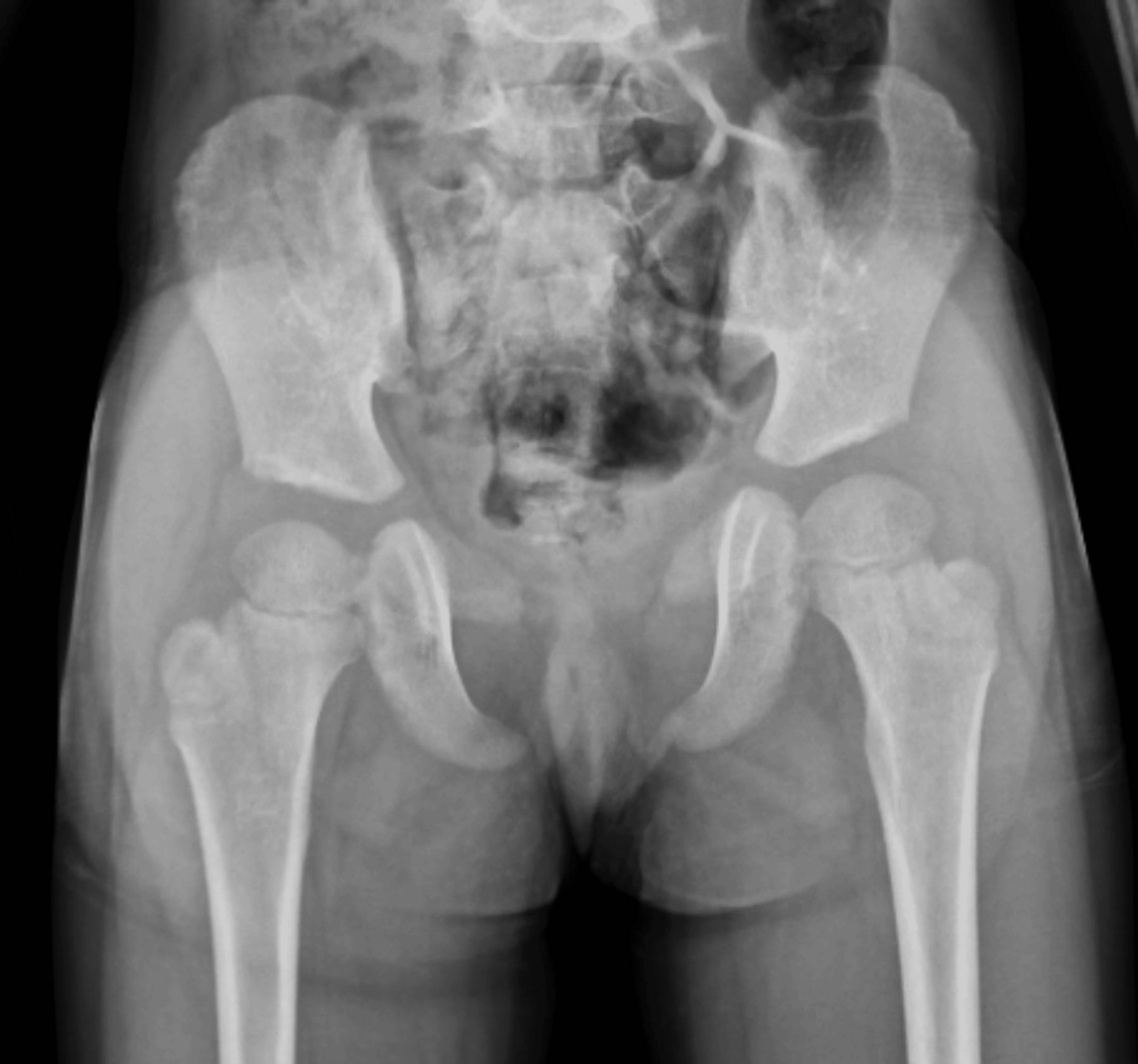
Frontal pelvic radiograph showing bilateral absence of the pubic bones with pseudo-widening of the pubic symphysis.
